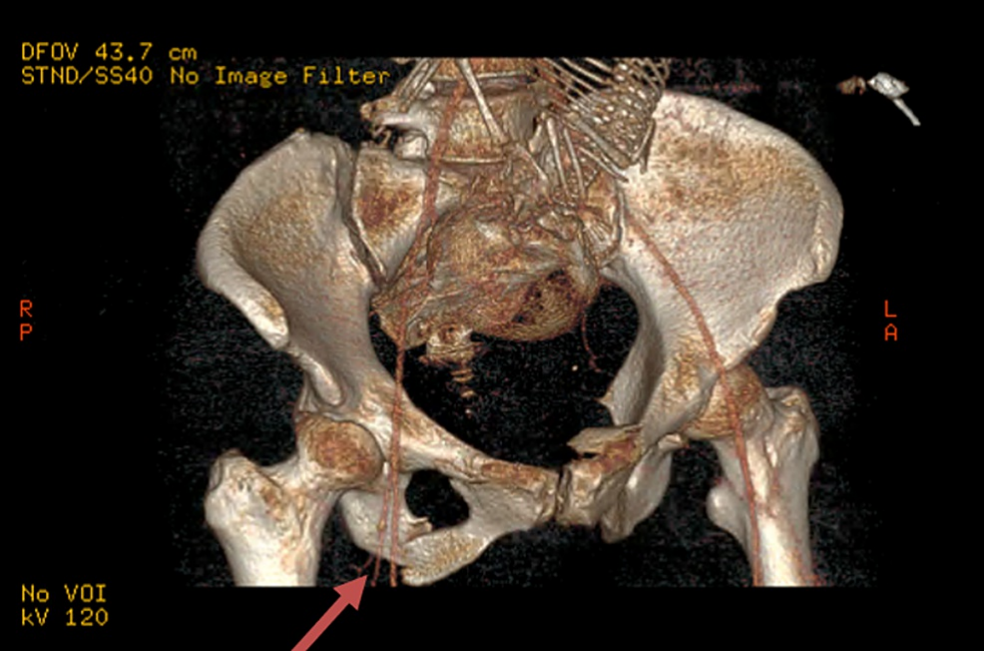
Pelvic CT - 3D reconstruction of the pelvic fracture (arrow)
